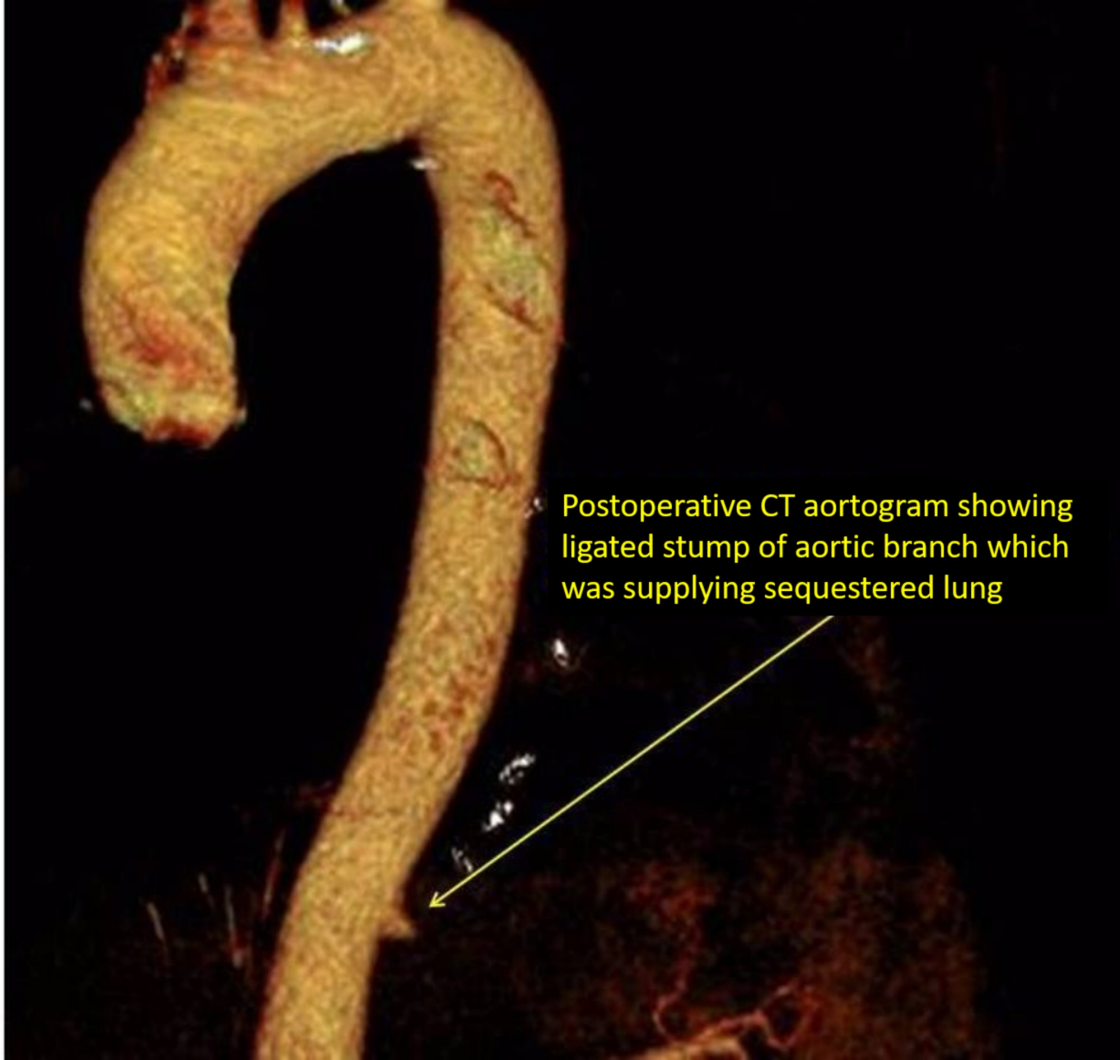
Postoperative CT aortogram showing the division of the feeding artery CT, computed tomography
